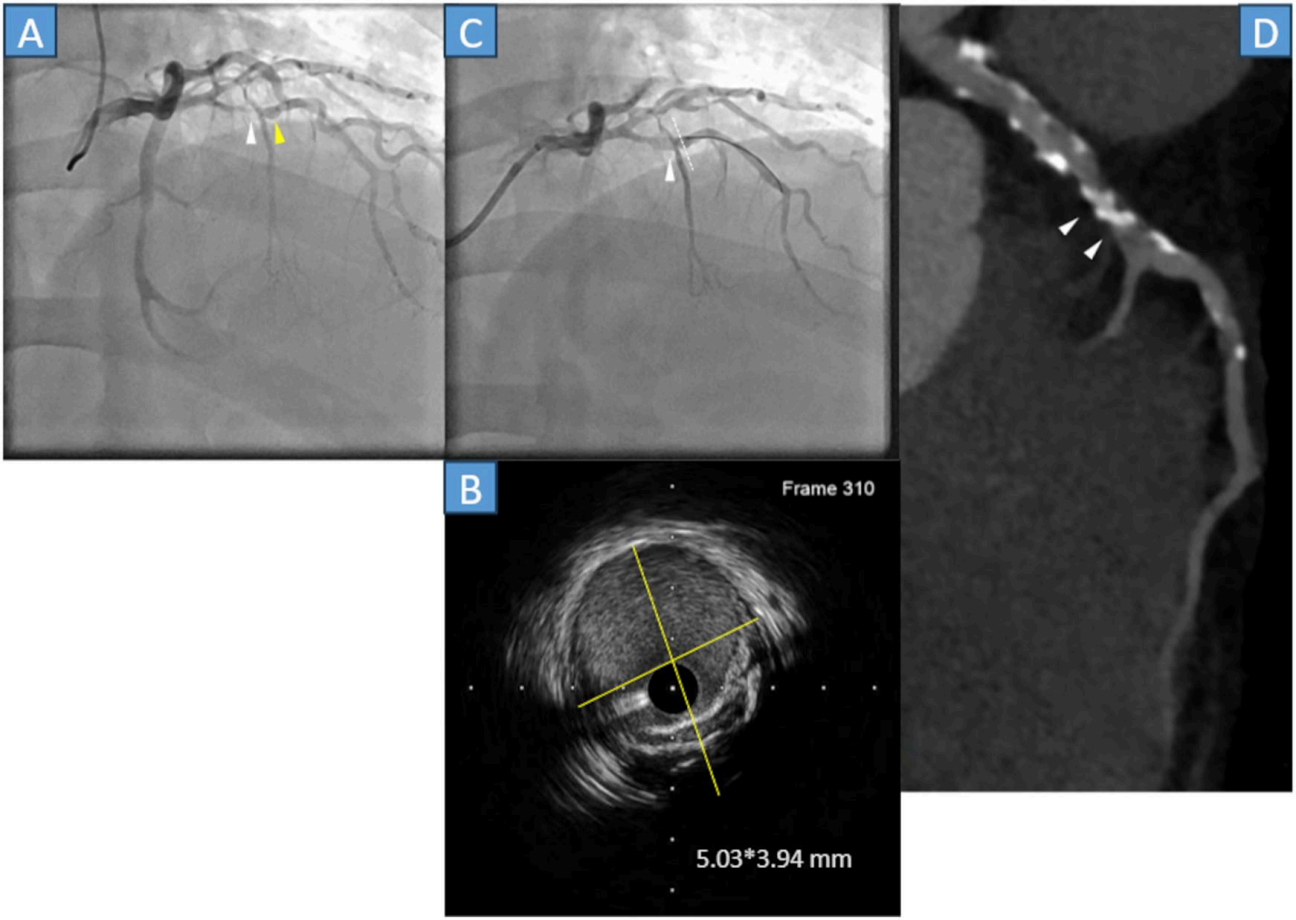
(A) Coronary angiography (CAG) showed stenosis just before the septal branch (white arrowhead) and aneurysm (yellow arrowhead). (B) The cross-sectional image of the intravascular ultrasound (IVUS) indicates a 5.03*3.94 mm aneurysm with calcification (yellow lines). (C) Final CAG reveals that the left anterior descending (LAD) artery was dilated with National Heart, Lung, and Blood Institute grade A dissection (white arrowhead). The white dots in the angiography correspond to the cross-sectional IVUS image. (D) Cardiac computed tomography indicates that the calcified lesion was patent (white arrowheads).
